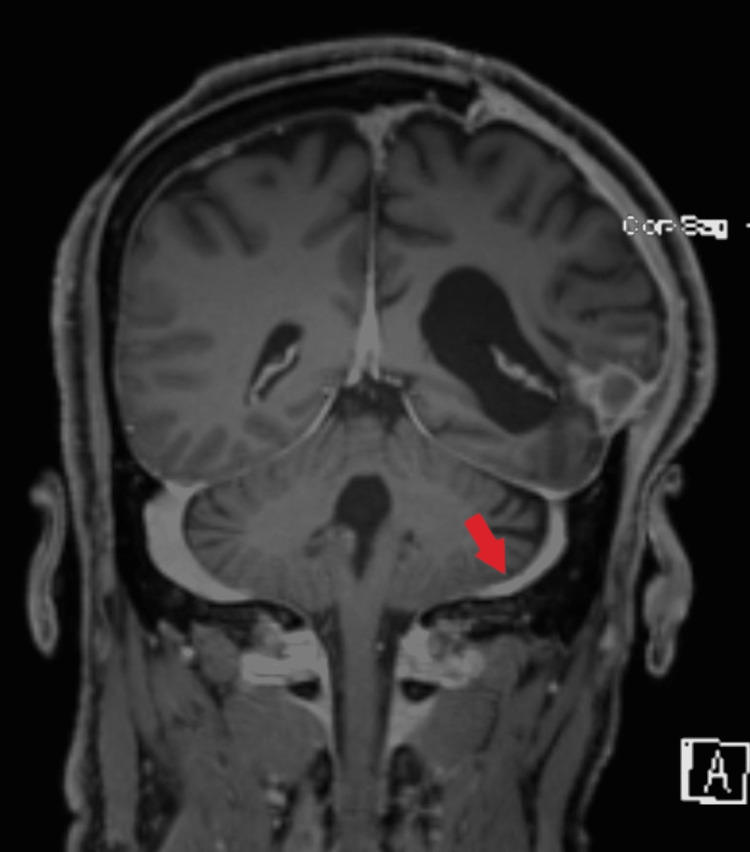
An MRI head: arrow showing re-canalization of the left sigmoid sinus.
